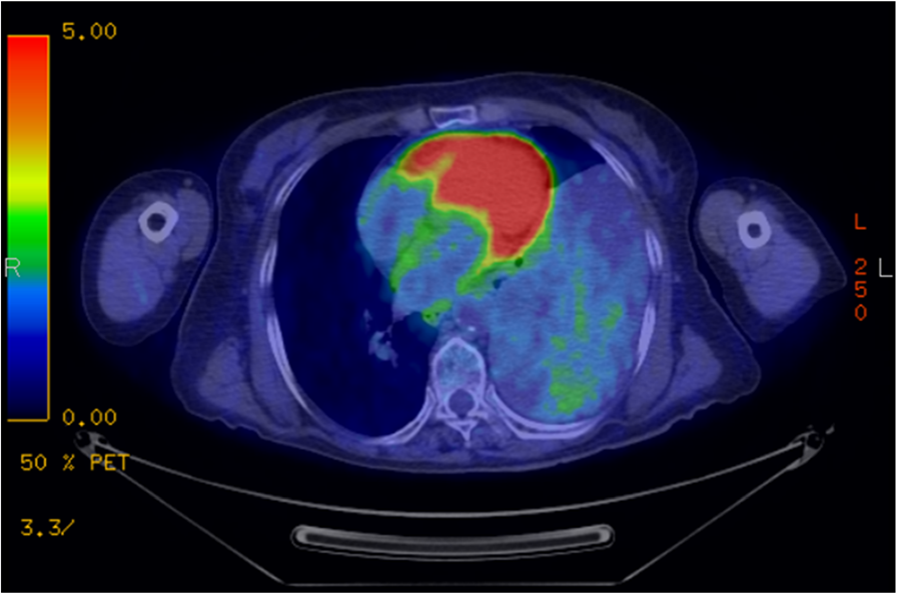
FDG-positron emission tomography shows low FDG uptake in the intrathoracic mass (a low SUV)
